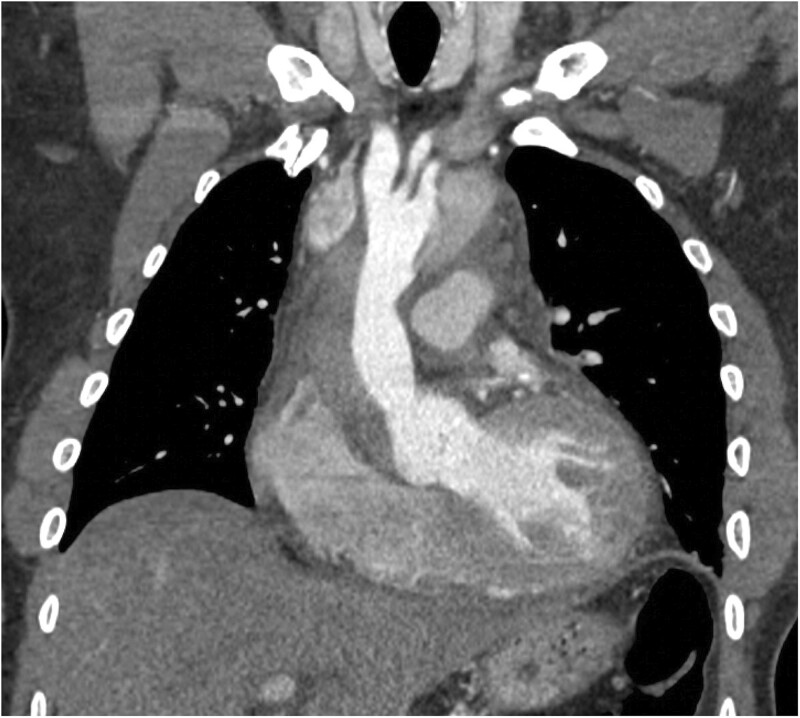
Coronal view of a contrast-enhanced CT in arterial phase demonstrating a retrograde type A aortic dissection with completely thrombosed proximal false lumen in the ascending aorta.
